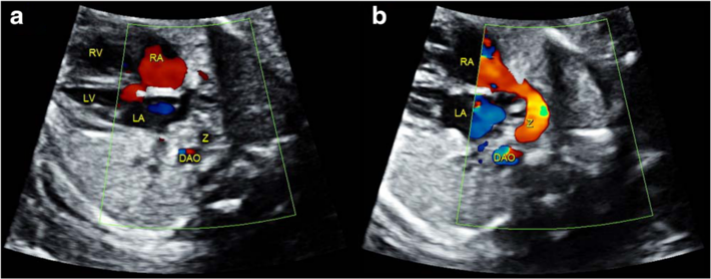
Sonographic images of a 22-gestational-week fetus diagnosed with interrupted IVC and azygos continuation. In the 4CV, a venous-like structure was detected adjacent to the DAO (a). Gradual rotation of the ultrasound beam; this structure was visualized to drain into the RA (b), and suspected to be the azygos. The abnormal structures were missed by sonographer under Protocol A, while they were detected under Protocol B as this sonographer knew that this sign was an indication of interrupted IVC and azygos continuation. 4CV, four-chamber view; DAO, descending aorta; LA, left atrium; LV, left ventricle; RA, right atrium; RV, right ventricle; Z, azygos
